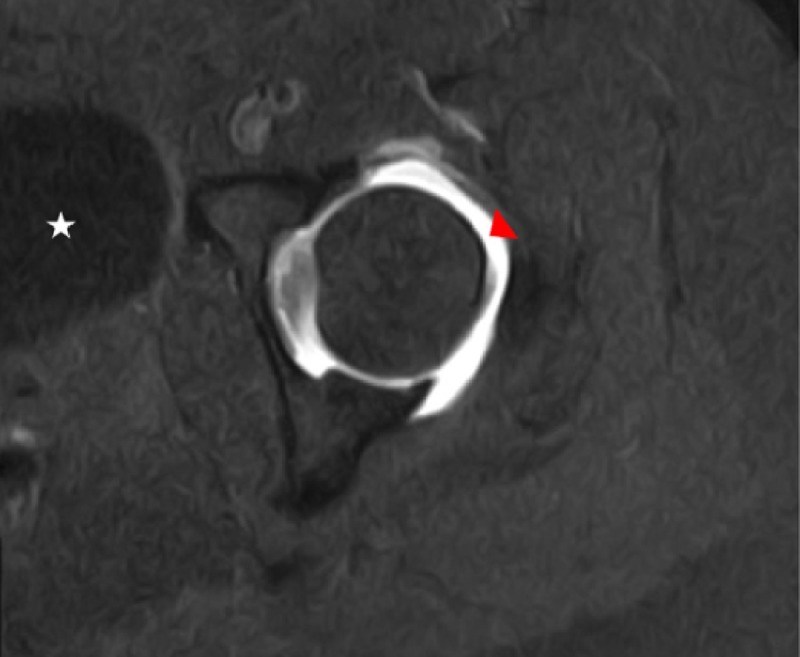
T1 weighted axial fat-saturated magnetic resonance image of the affected left hip A repeated T1 weighted axial fat-saturated magnetic resonance image of the affected left hip status post intraarticular injection for magnetic resonance arthrography is shown. The hyperintense effect of correctly diluted gadolinium (red arrowhead) in comparison to the hypointense appearance of the partially imaged urinary bladder (star) is also demonstrated.
